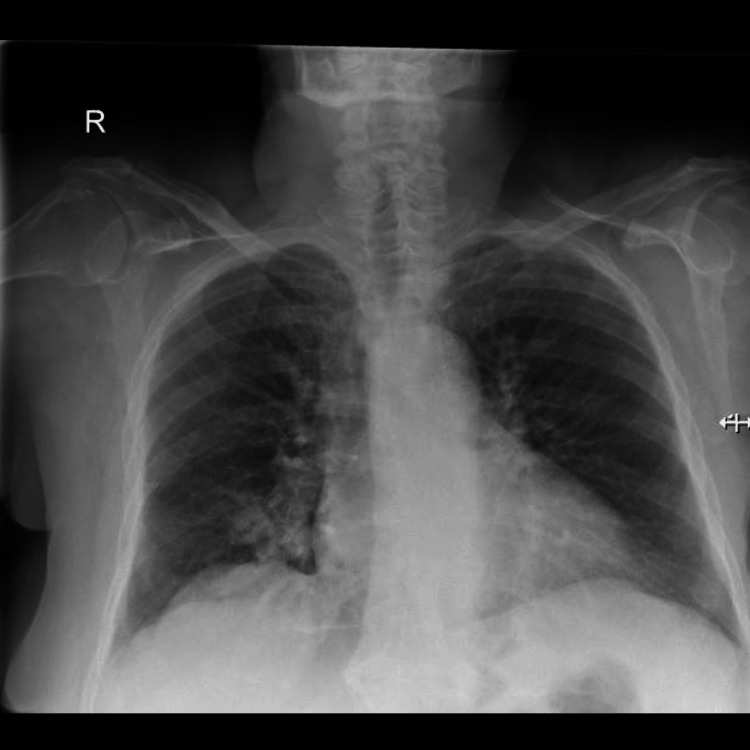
The repeated PA chest X-ray where the curvilinear lines are no longer apparent, ruling out the presence of pneumothorax. PA: postero-anterior
